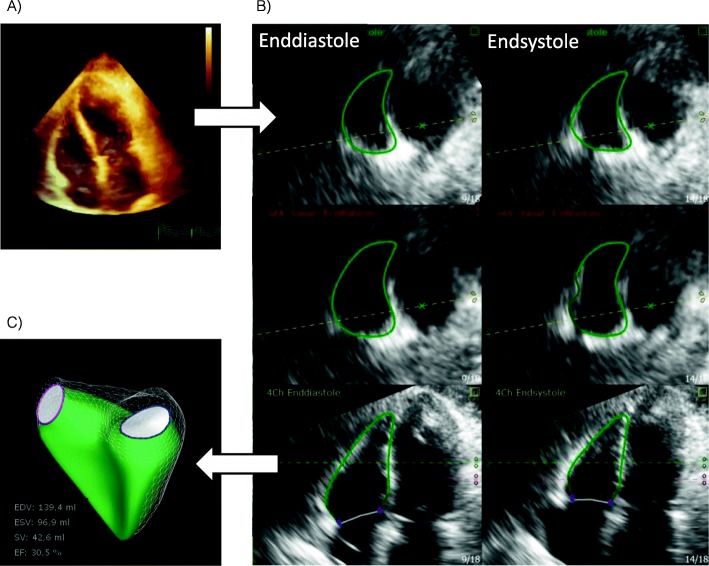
a 3D full volume dataset of the left and right ventricle based on an apical 4-chamber view. b Endocardial border tracking by the Tomtec 4D RV-function package. The first column shows the enddiastolic frame, the second column shows the endsystolic frame. The First and second lines show medial and basal transversal planes of the RV. The third line shows the right ventricle in a reconstructed apical view. c Results of the Tomtec 4D RV-function package showing the volumetric model of the RV. The mesh grid shows the enddiastolic volume, the green inner model shows the endsystolic volume. Absolute values of volumes and the ejection fraction are also reported
